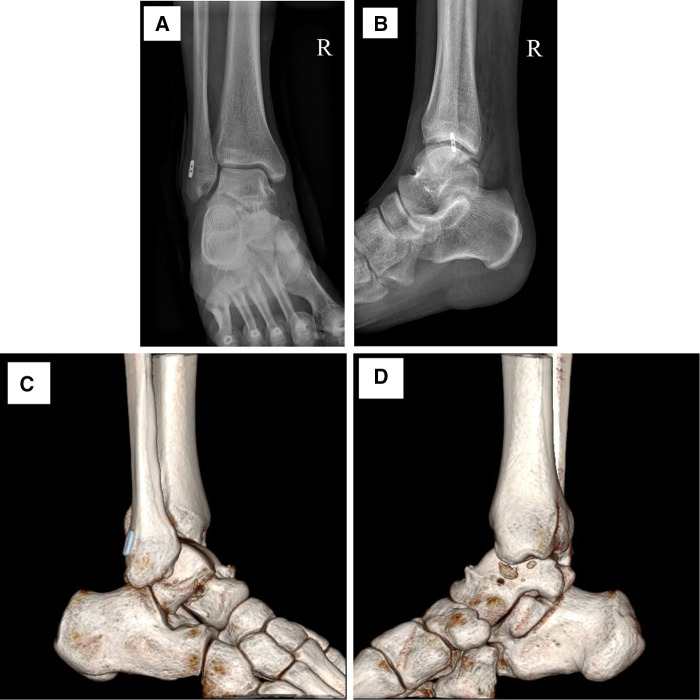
A typical case of the anterior talofibular ligament injury after anatomical reconstruction with autologous half-bundle peroneal longus tendon. (A) Post-operative X-ray image in the anterior position. (B) Post-operative X-ray image in the lateral position. (C,D) Post-operative CT reconstruction image in the sagittal position.
